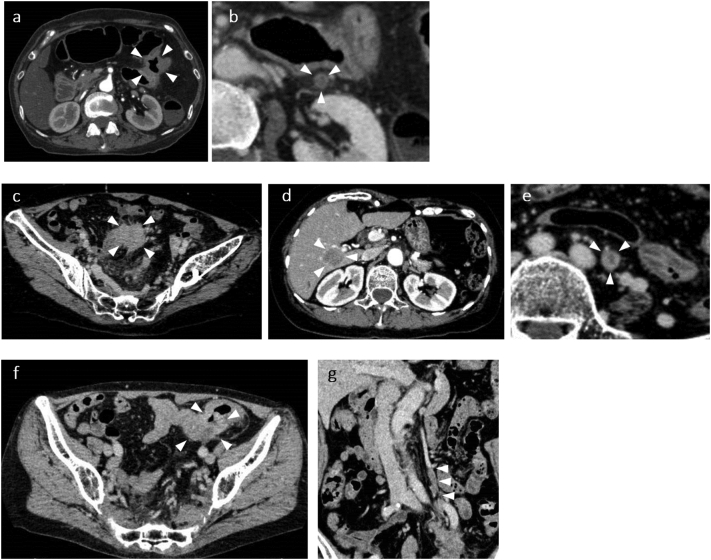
CT scan findings. a) Circumferential wall thickening with contrast enhancement was observed in the left transverse colon in Patient 1. b) IMV tumor thrombosis was suspected due to poor contrast and dilation of the IMV in Patient 1. c) Circumferential wall thickening with contrast enhancement was observed in the rectosigmoid colon in Patient 2. d) A mass lesion with irregular ring enhancement was found in liver (S6) in Patient 2. e) IMV tumor thrombosis was suspected due to poor contrast and dilation of the IMV in Patient 2. f) Circumferential wall thickening with contrast enhancement was observed in the sigmoid colon in Patient 3. g) IMV tumor thrombosis was suspected due to poor contrast and dilation of the IMV in Patient 3.
